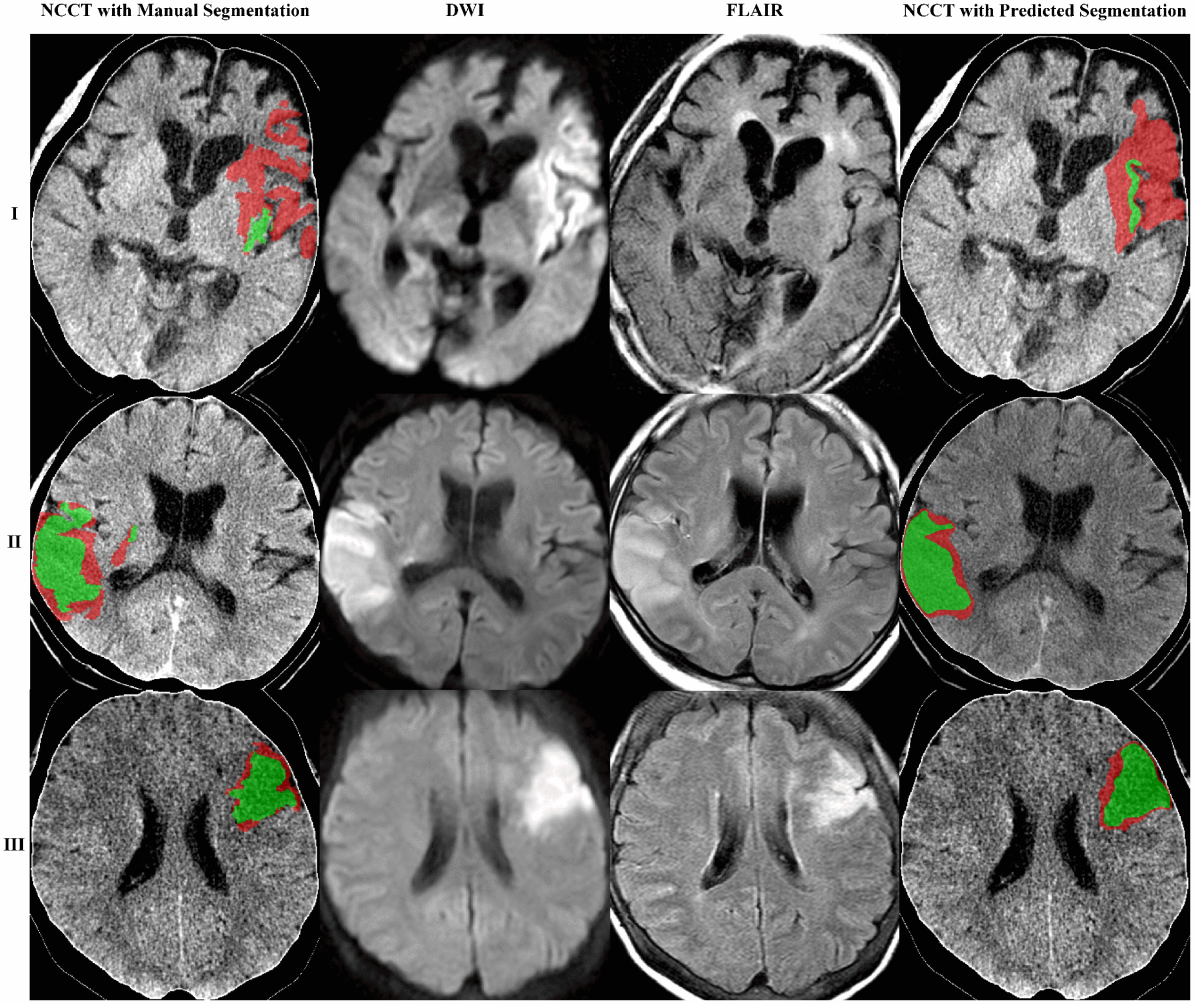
The proposed model obtained three segmentation examples. The images are shown as NCCT overlaid with the manually or algorithm-segmented DWI and FLAIR lesions colored in red and green, respectively. Case example of mismatch (I) and no mismatch (II and III).
